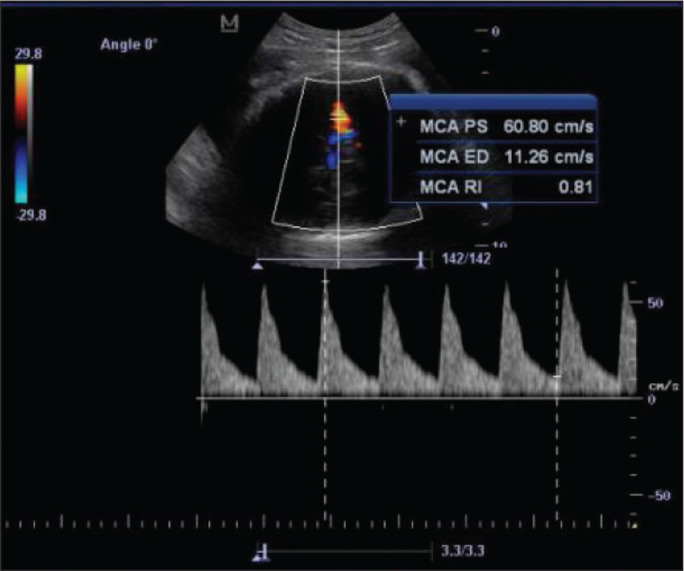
Color-flow sonogram of the MCA showing the typical biphasic flow.
